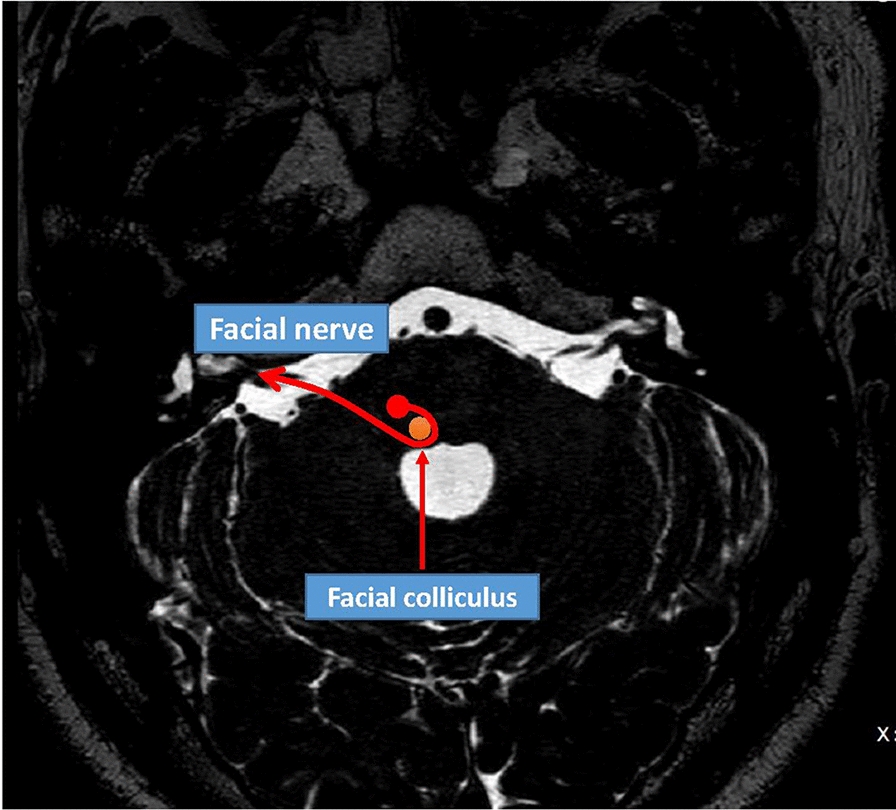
Facial colliculus of MRI: T2-weighted MRI image at the level of the facial colliculi demonstrates the course of the facial nerve within the pons. Note the route of the facial nerve with a loop around the abducens nerve nucleus (saffron one)
